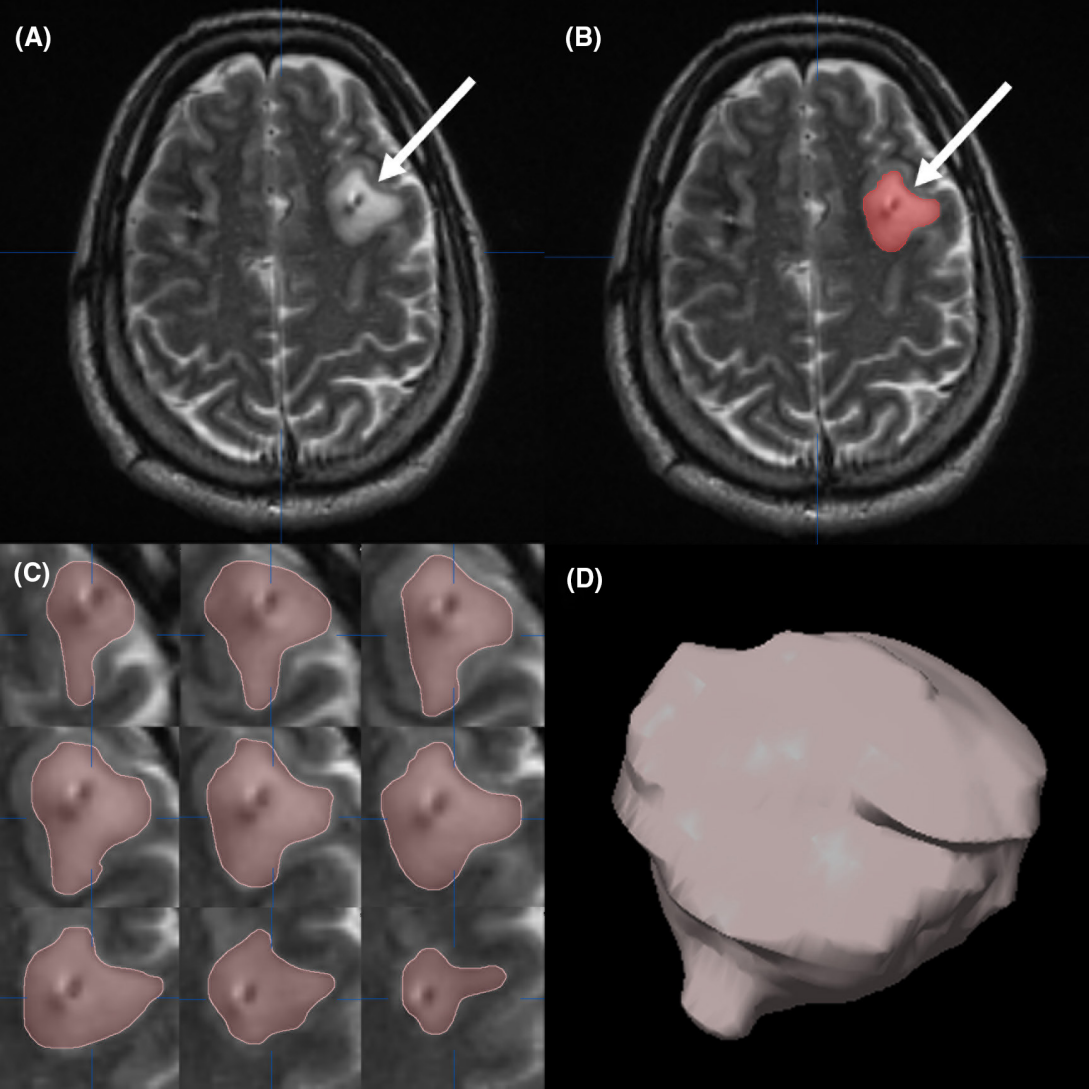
Measurement of volume of peri‐electrode edema. (A) Peri‐electrode edema on T2‐weighted MRI (the white arrow). (B) A mask covering the signal hyperintensity area was manually created on the slice (the white arrow). (C) The masks covering all signal hyperintensity areas alongside the electrode were segmented on each slice. (D) A three‐dimensional object representing the peri‐electrode edema was created based on the masks and volumetric estimation was performed using the software.
